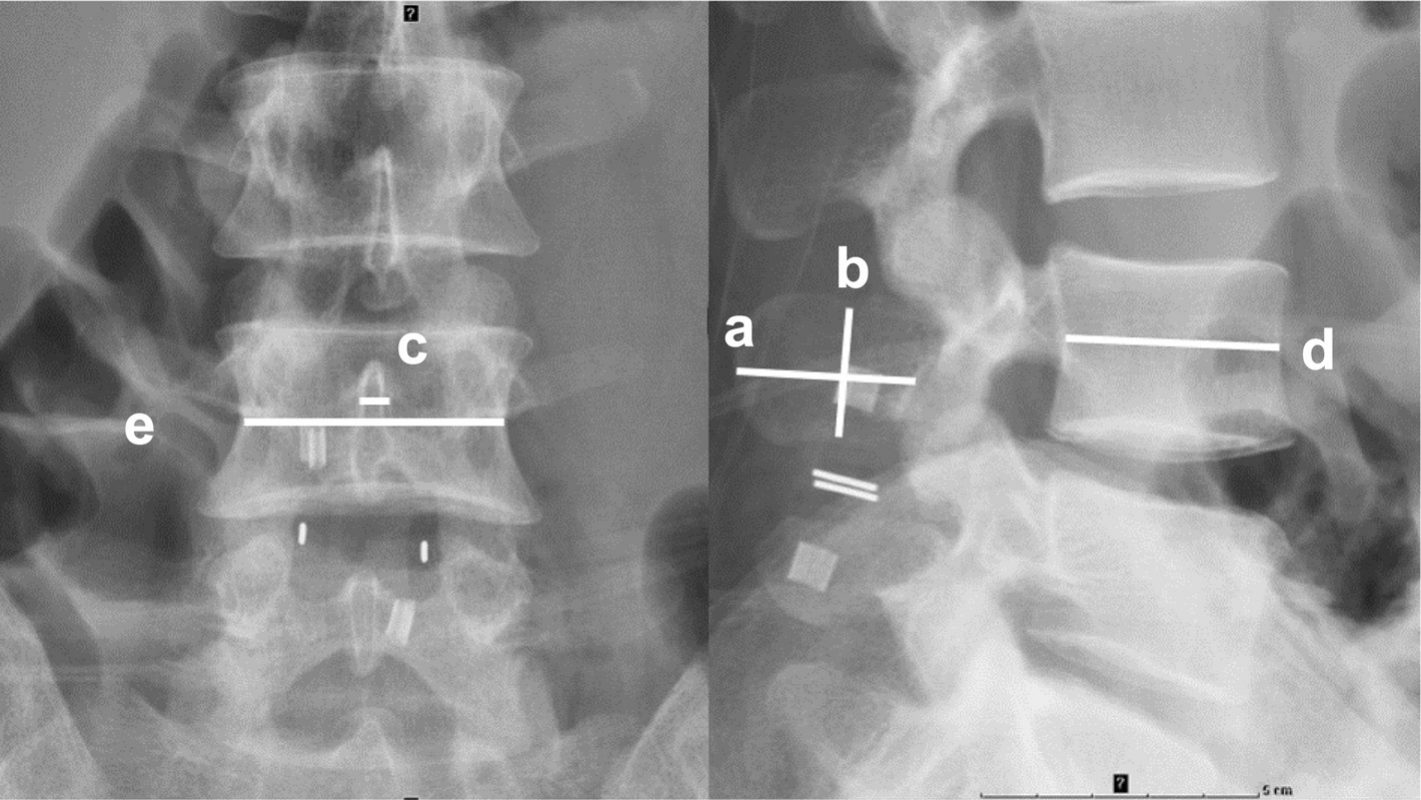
Radiographic measurement. Right: Later view. Left: Anteroposterior view. a=Length of spinous process. b=Height of spinous process. c=Width of spinous process. d=Length of the L4 vertebrae. e=Width of the L4 vertebrae.
